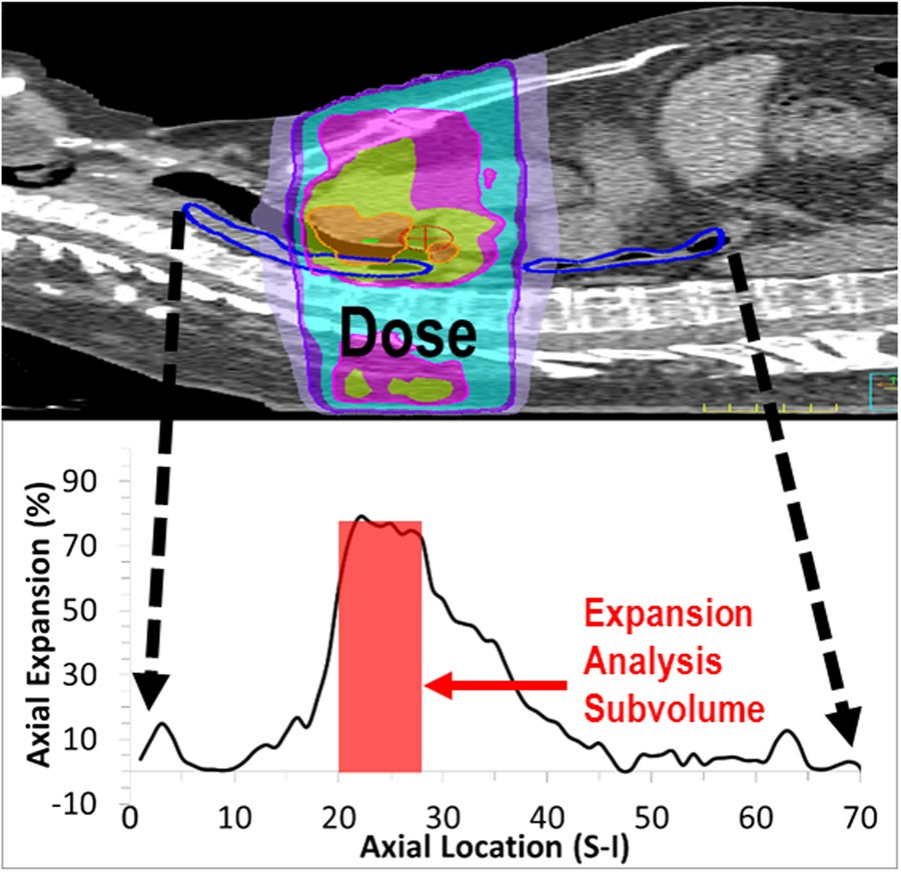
An example of esophageal expansion. (Top) Example patient thoracic CT with a colorwash of the radiation therapy dose distribution and esophageal segmentation (blue). (Bottom) Axial profile of esophageal expansion calculated at along the superior-inferior direction of the esophagus. The shaded red box is the esophageal subvolume region of analysis. Note the high expansion in the corresponding region of high radiotherapy dose.
